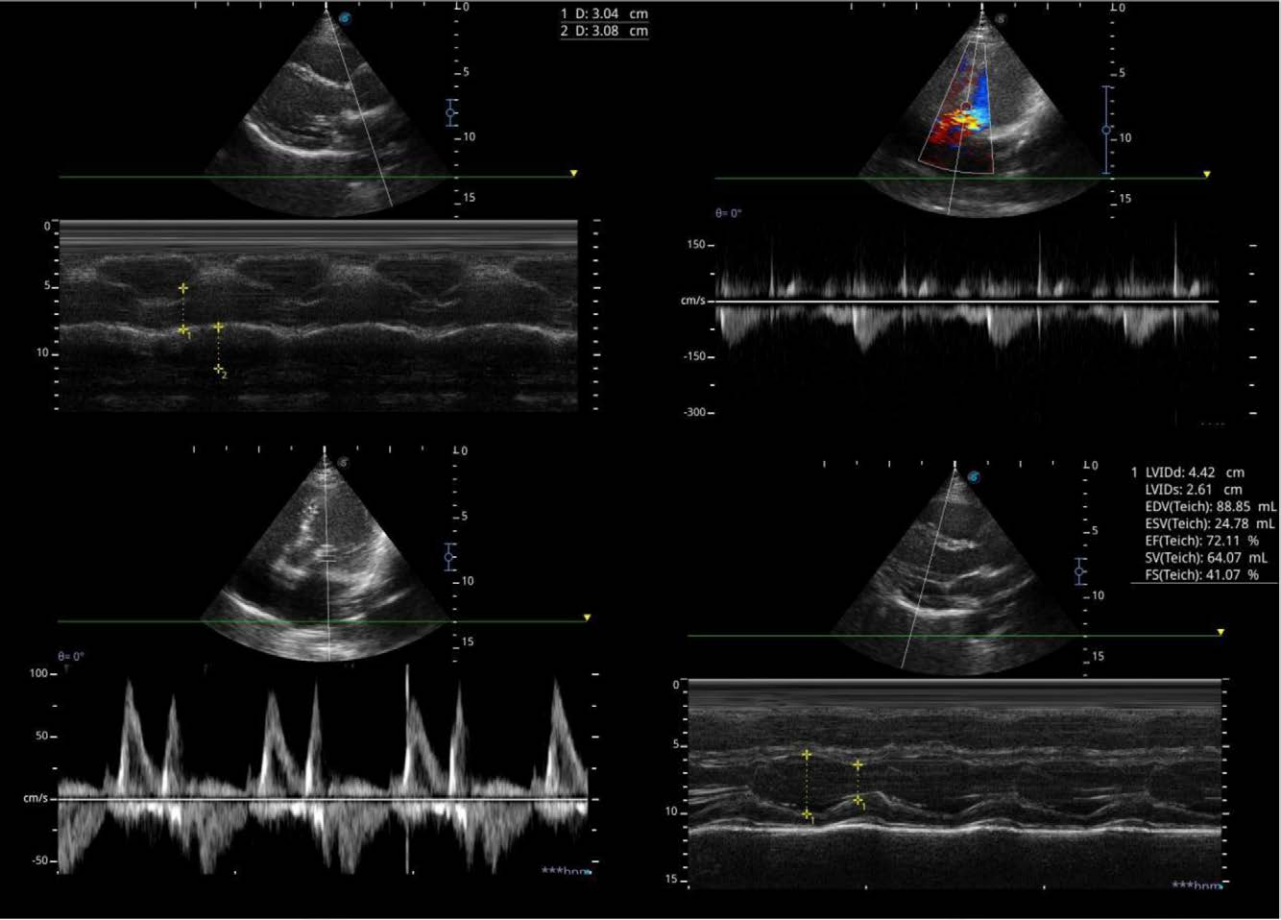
Regular follow-up echocardiogram showing no adverse effects on the cardiac function.
